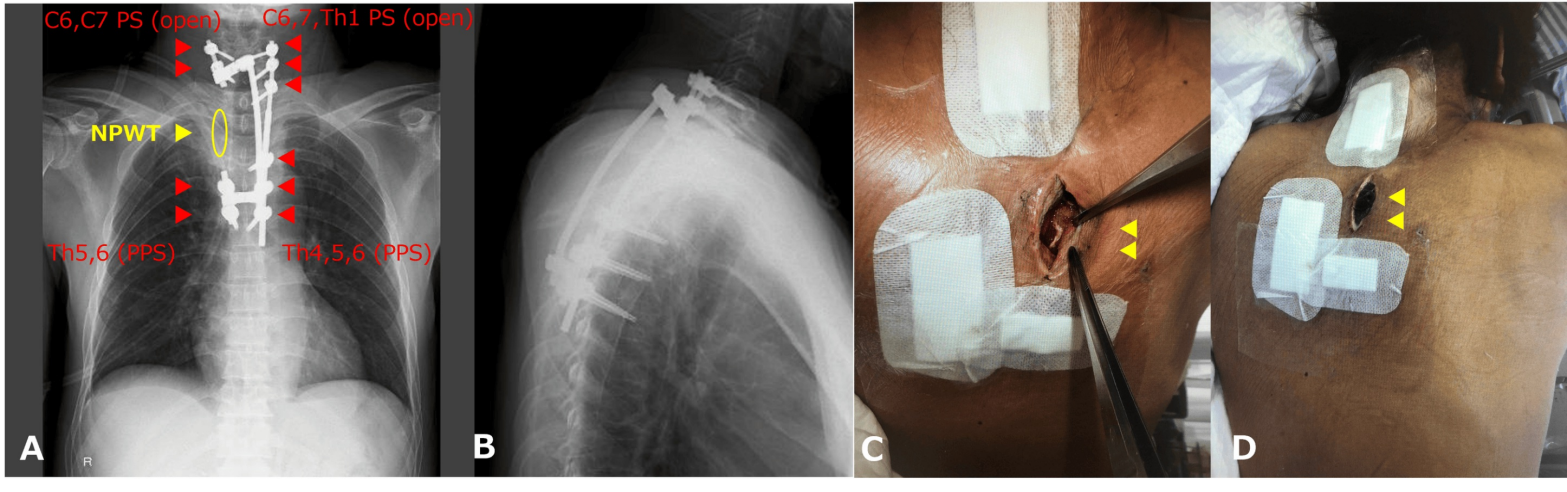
Postoperative images and clinical photographs (A and B) Postoperative anteroposterior and lateral radiographs are shown. For NPWT, a foam sponge was placed in the area circled in yellow. (C) Skin incision area with drainage (arrowheads). (D) A foam sponge for NPWT was placed on the skin incision (arrowheads). NPWT: negative pressure wound therapy
